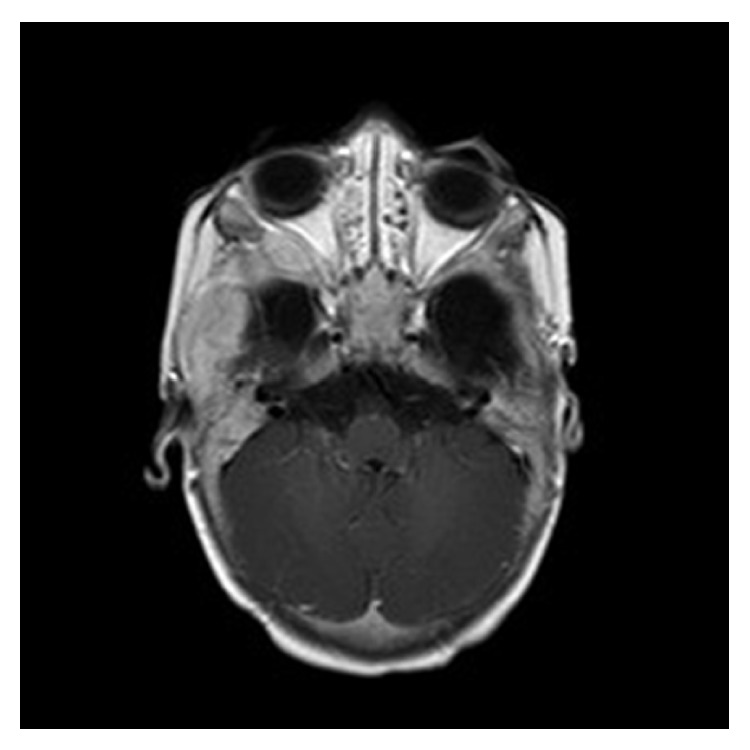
MRI of face showing soft tissue mass in the left mastoid region with osteolytic lesion in the greater wing of sphenoid bone, left temporal, and right temporal regions.
